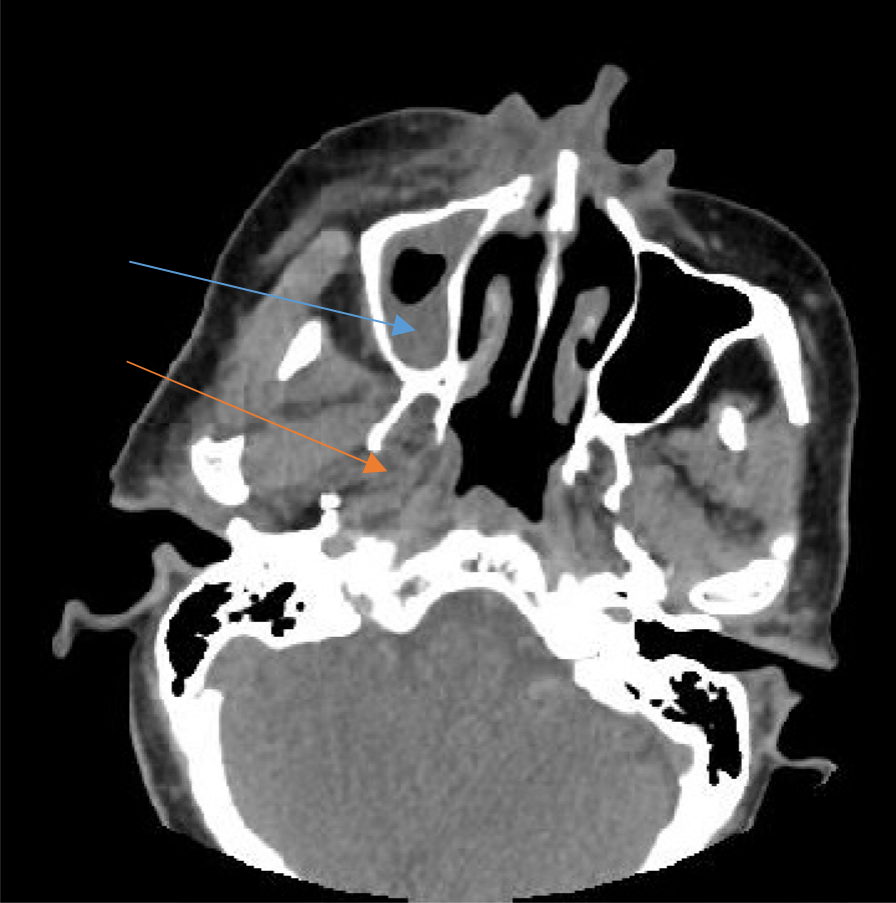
Axial section of plain CT of the brain in a patient with sinonasal mucormycosis showing mucosal thickening of the right maxillary sinus (blue arrow) with involvement of the right pterygopalatine fossa (red arrow)
